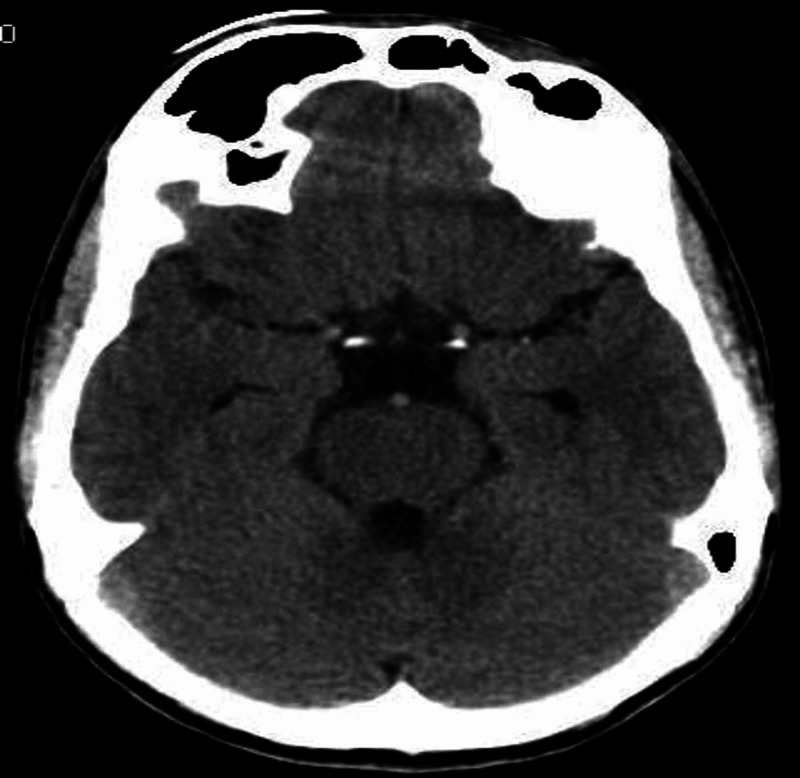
CBCT axial slice showing depressed fracture of left supraorbital bone involving frontal sinus.
